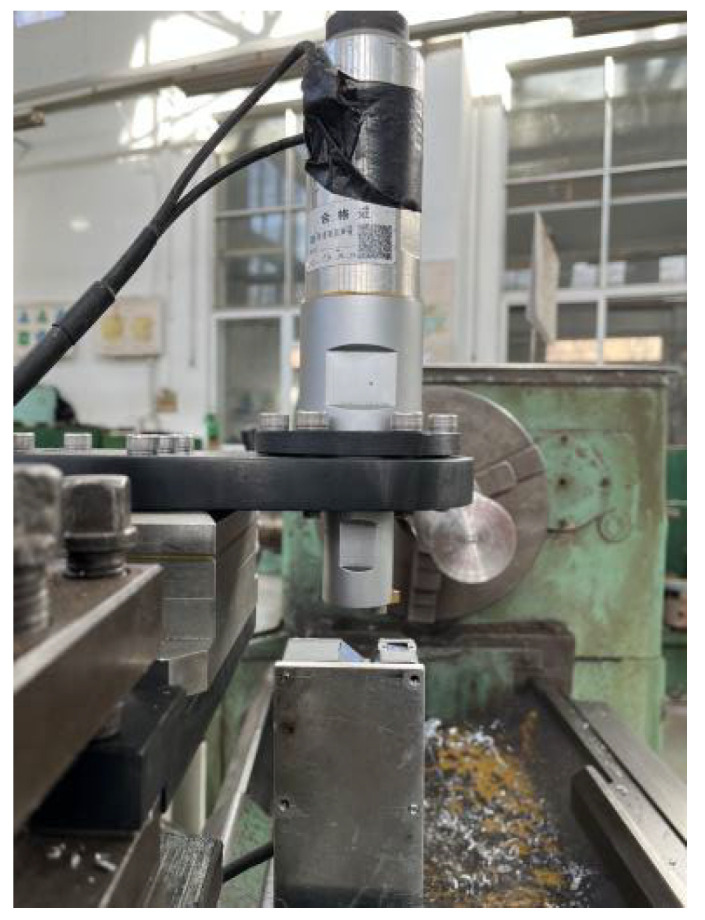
Point laser measurement of ultrasonic amplitude.
